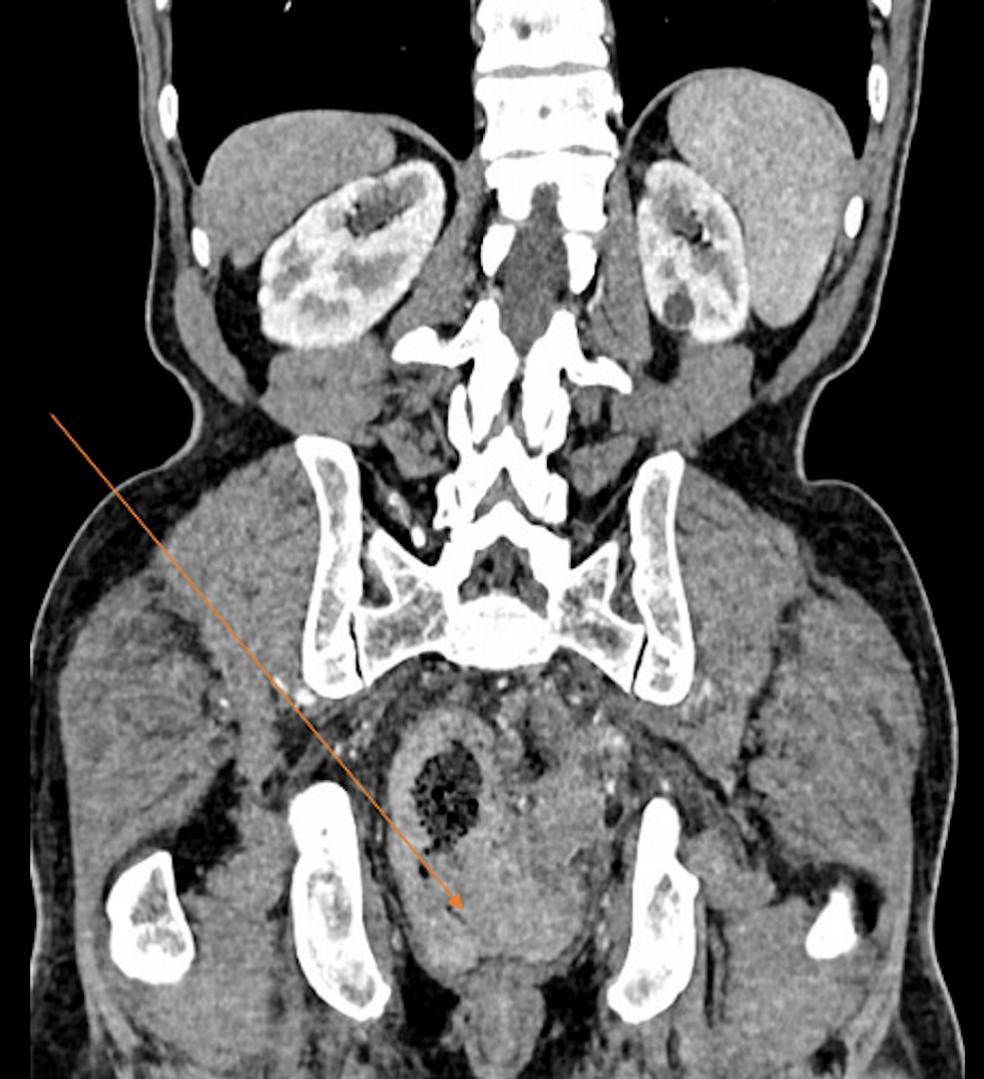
Coronal section of contrast CT scan of the abdomen. The arrow denotes circumferential thickening of the segment of the sigmoid colon causing a stricture and upstream dilatation of the loop of the colon. The lesion extends beyond the serosa and invades the adjacent rectum.
